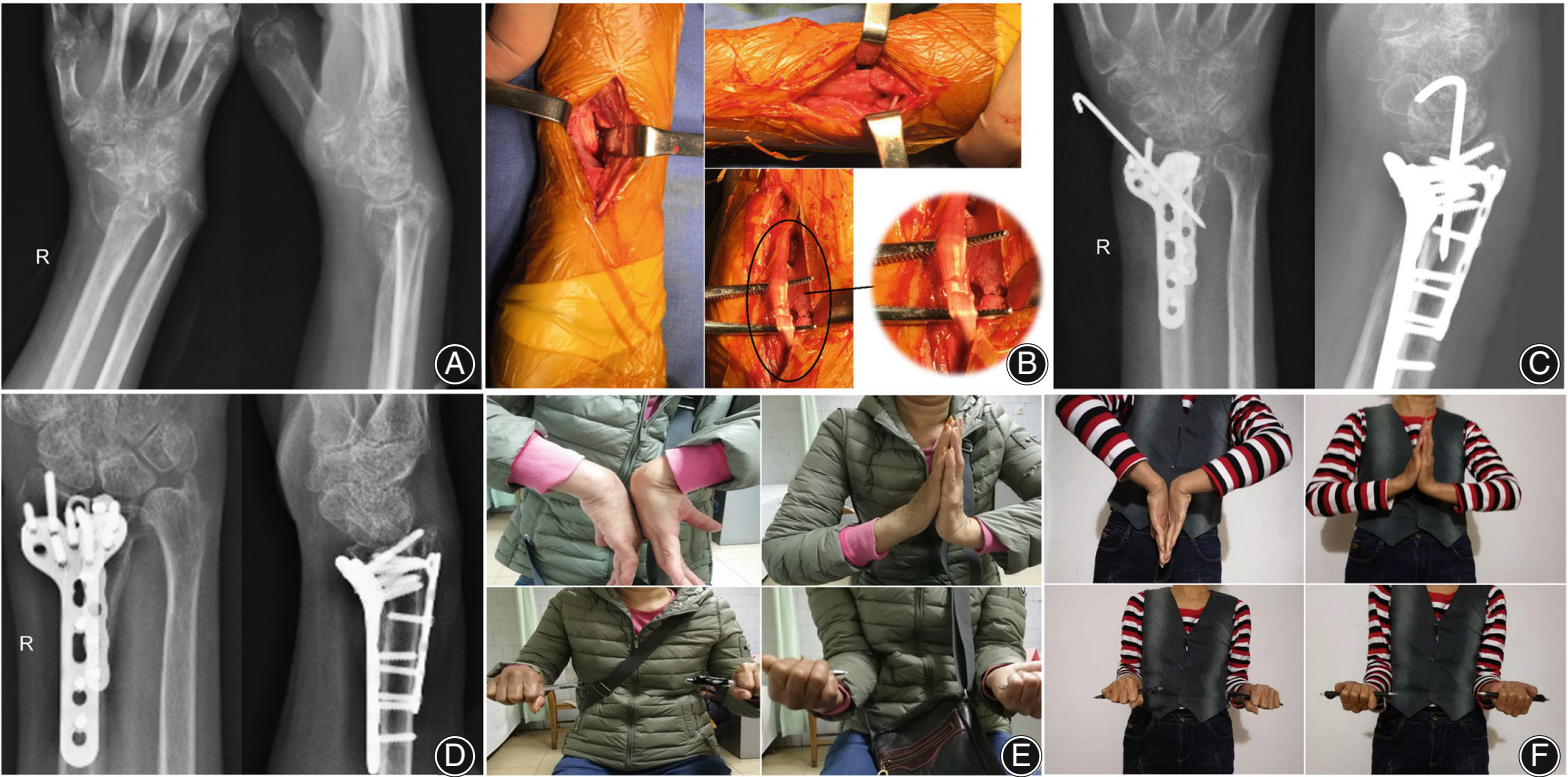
A 58‐year‐old woman suffered from malunion of distal radius fractures and a shortened radius. (A) Anteroposterior and lateral radiographs of the right radiocarpal joint. (B) Corrective osteotomy was performed along the original fracture line from the volar and dorsal approaches. The extensor pollicis longus tendon was inserted into the fracture site, and a part of it was damaged. (C) Corrective osteotomy with volar and dorsal plate fixation restored radius length and volar tilt. Anteroposterior and lateral radiographs at 3 months postoperatively. (D) Anteroposterior and lateral radiographs of a slightly shorter radius length at 18 months postoperatively. (E) Wrist function was evaluated by flexion and extension of wrist and pronation and supination of the forearm at 3 and (F) 18 months postoperatively
